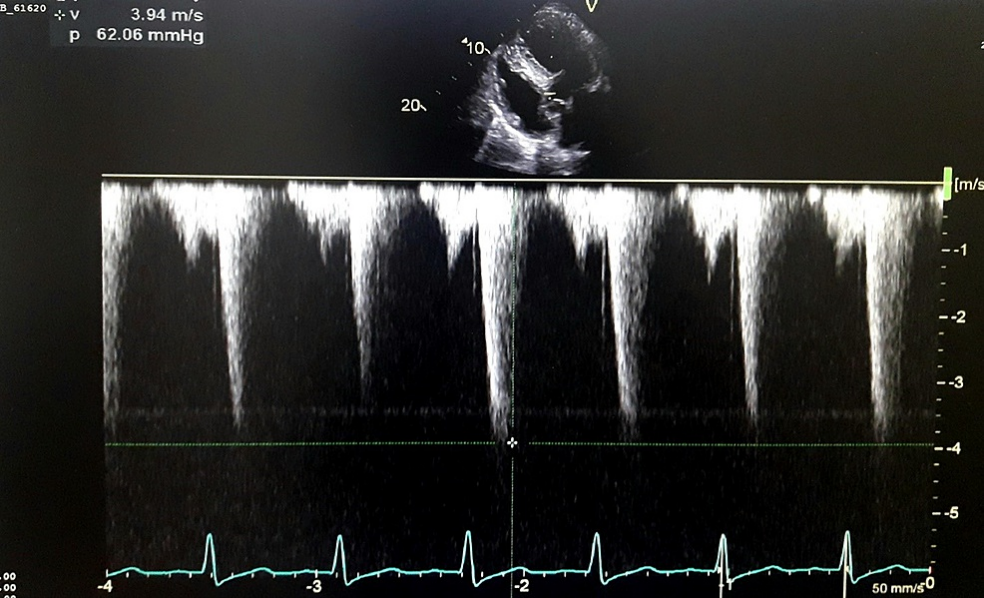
Echocardiography showing a complete SAM of the AML causing severe LVOT obstruction, with a peak gradient of 62 mmHg SAM: subaortic membrane; AML: anterior mitral leaflet; LVOT: left ventricular outflow tract
